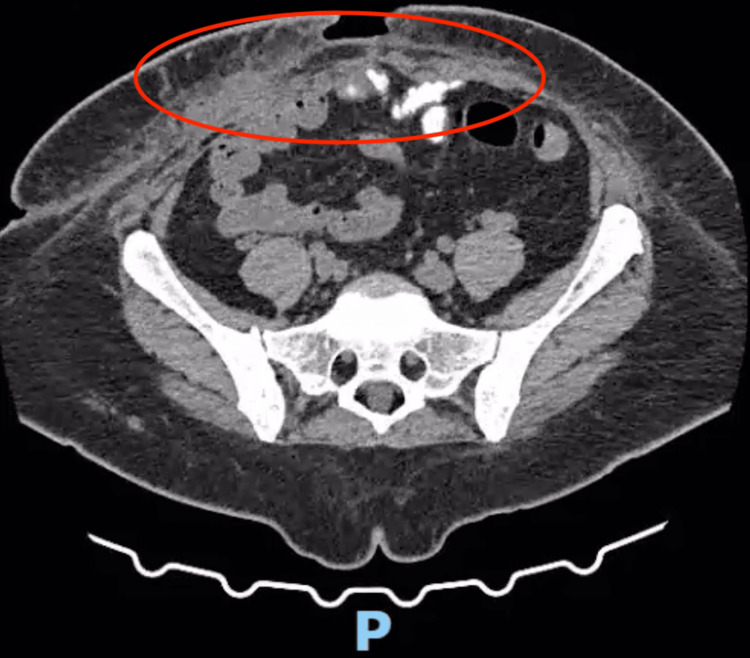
CT scan one year after surgery no wall defect (red circle) CT: computed tomography
